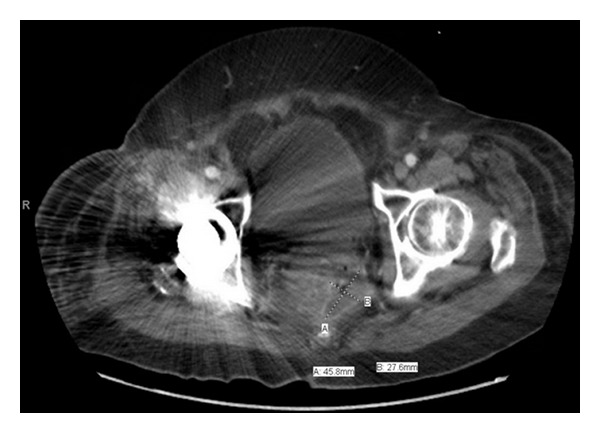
CT scan of the pelvis showing a perirectal fluid collection containing air locules. Fistulous tract to the anorectum not seen in this section.
